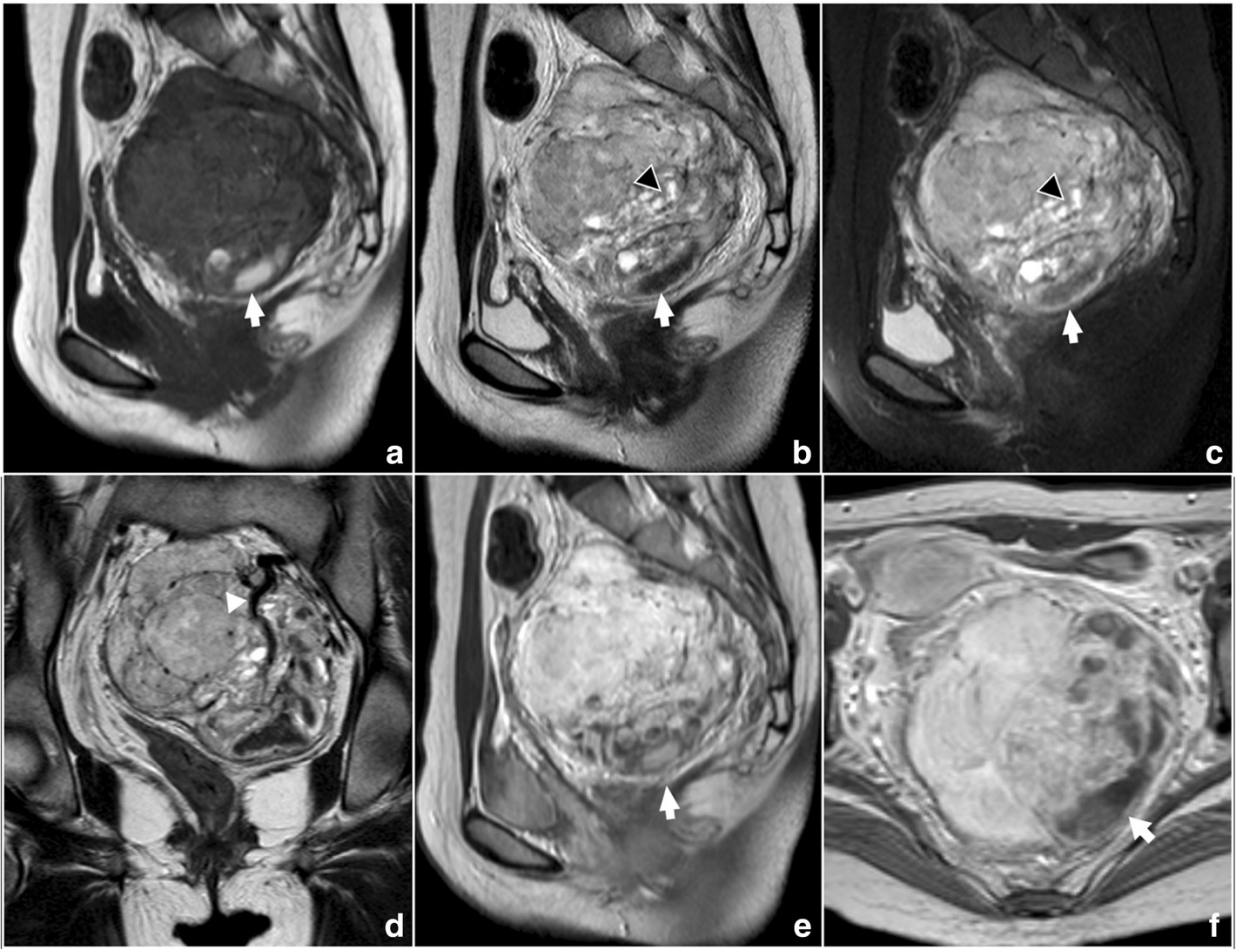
MRI examination of the pelvic cavity. Sagittal T1-weighted image (a) shows a well-defined lobulated mass with isointense and slight hypointense in the pelvic retroperitoneum. The lower part of the mass shows some areas with hyperintense (arrow). Sagittal T2-weighted image (b) and T2-weighted image with fat saturation (c) show the mass with inhomogeneous isointense and hyperintense. Cystic degeneration could be detected in the inner part (arrowhead). The lower part of the mass showed some areas with hypointense (arrow). Coronal T2-weighted image (d) shows large vessels with flow-void sign (arrowhead). Post-contrast T1-weighted images (e-f) show the mass with intense heterogeneous enhancement. The lower part with hyperintense on T1-weighted image and hypointense on T2-weighted image shows no significant enhancement (arrow)
